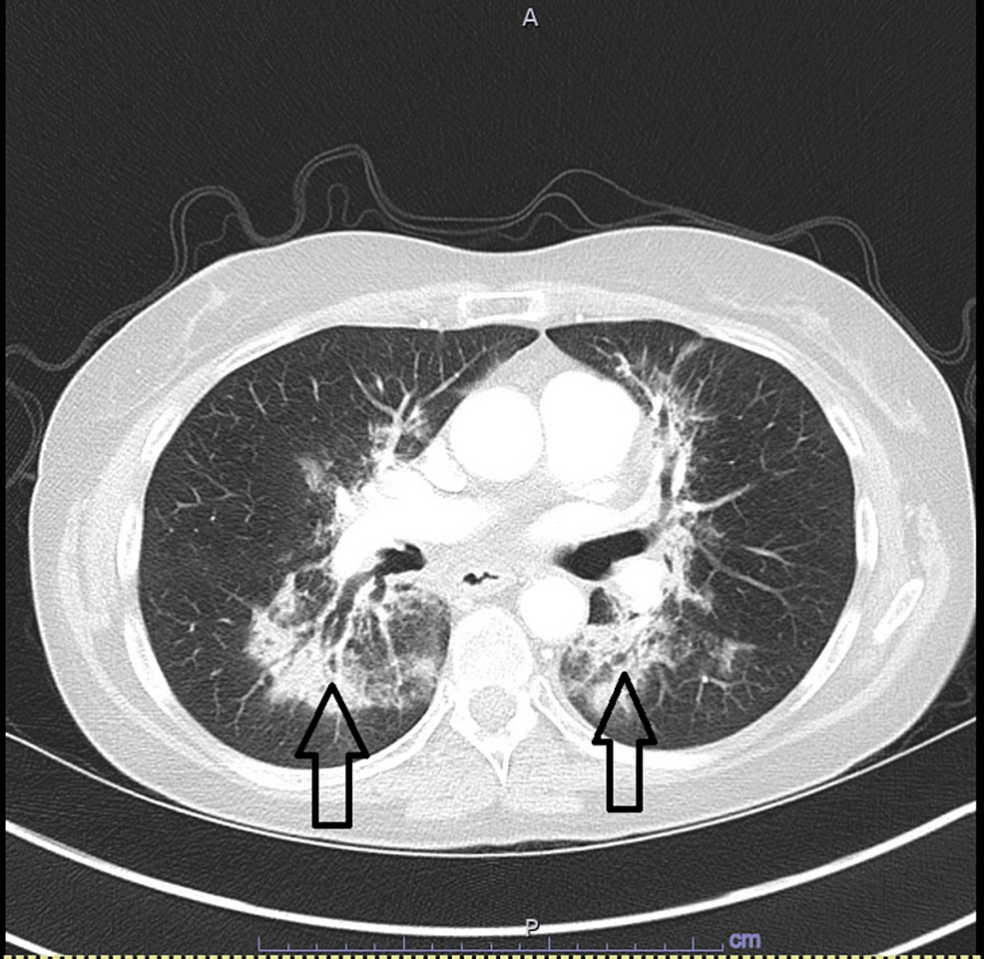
Chest CT Scan CT chest that shows the progression of diffuse bilateral ground glass opacities (arrows).
